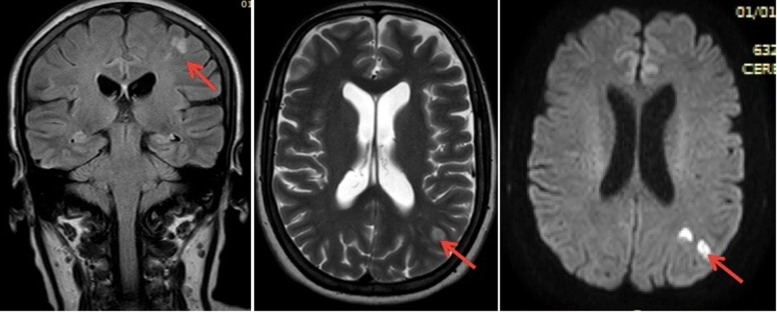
cerebral MRI revealing an acute ischemic stroke constituted on the left: cortico-subcortical area in the hyper signal
